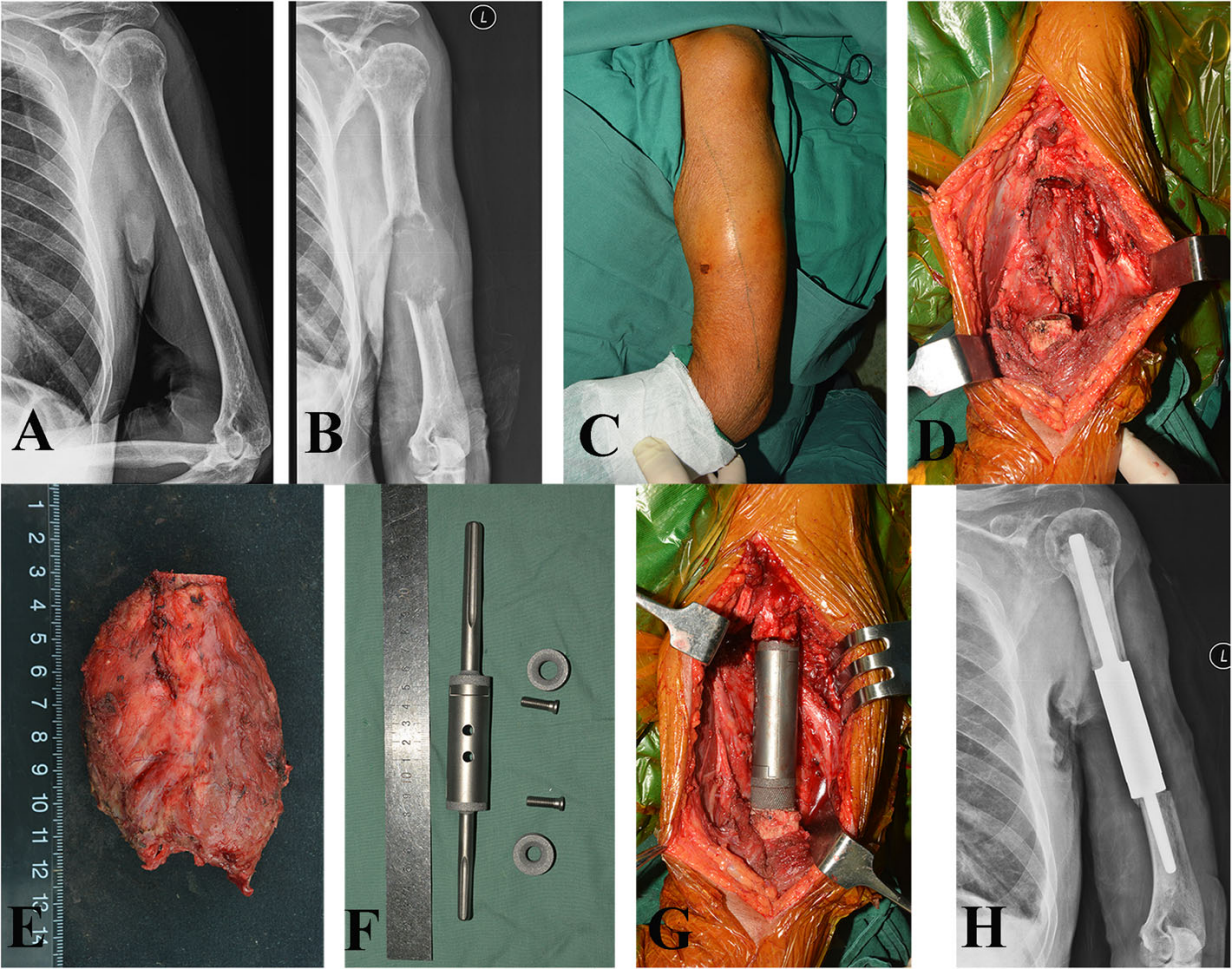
Intercalary prosthetic reconstruction for a pathologic fracture of the left humeral shaft in patient 59, a 76-year-old male. a Anteroposterior radiograph of the left humerus showed osteolytic destruction in the middle 1/3 of the humerus. b The metastatic lesion of lung carcinoma was enlarged despite 3 months of conservative treatment. c The incision was made proximally through a deltopectoral approach and distally on the arm over the medial border of the biceps muscle. d, e Intraoperative photos show that the lesion was resected widely. f, g Intraoperative photos show the custom-made prosthesis. h Postoperative X-ray images show that the prosthesis is in a good position
